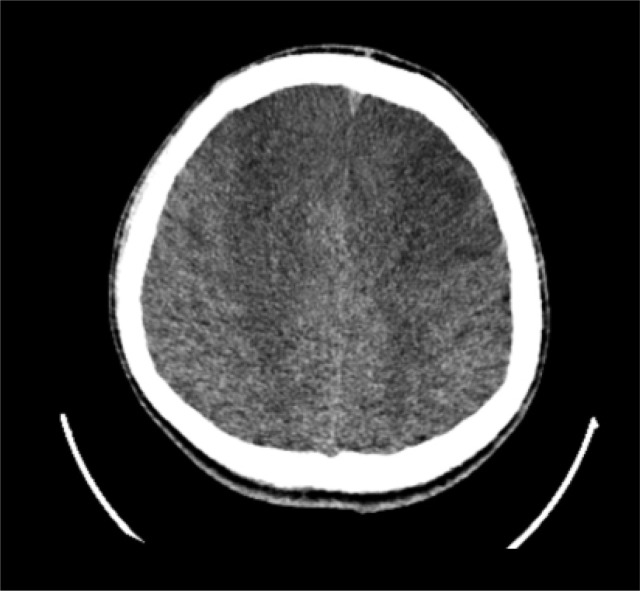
Computed tomography scan of the patient's brain obtained on the 8th day of admission.
